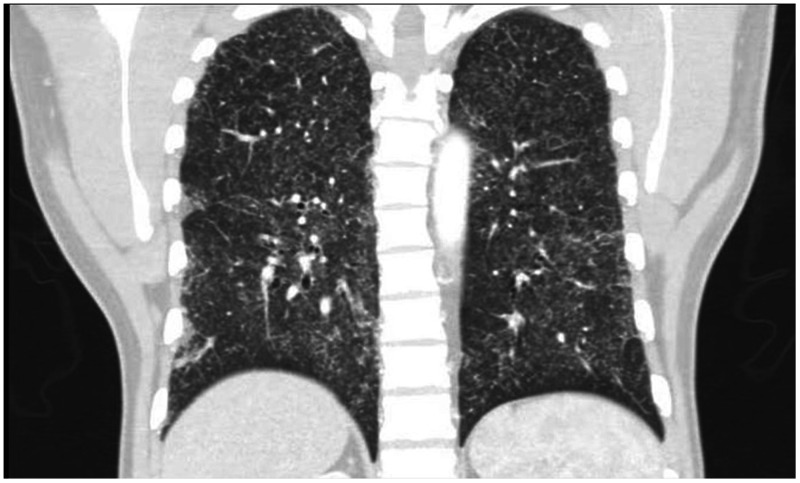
Coronal view. Contrasted chest computed tomography scan showing multiple centrilobular micronodules, with a budding tree pattern and ground glass.
